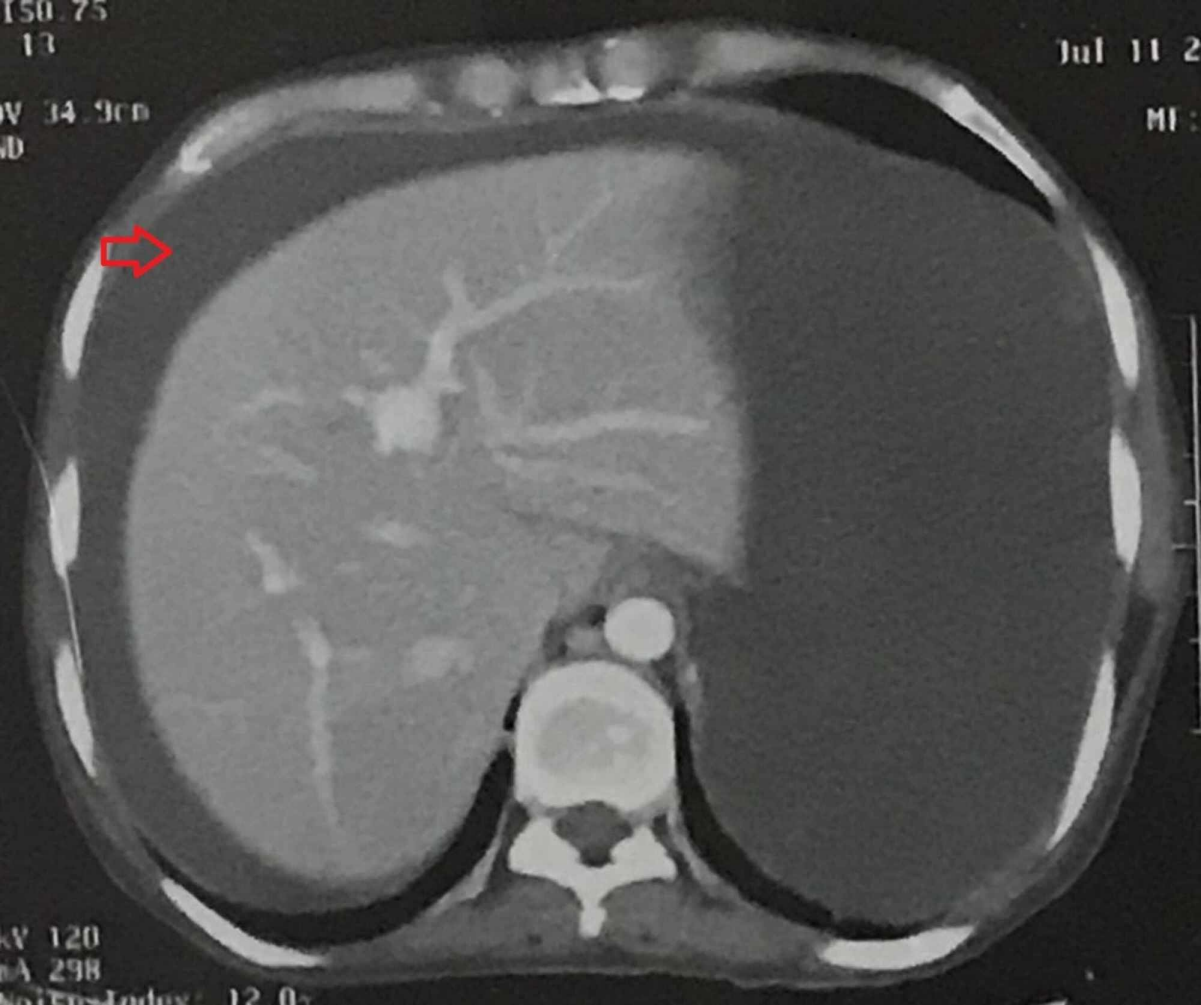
CT scan of the abdomen showing significant gross ascites
